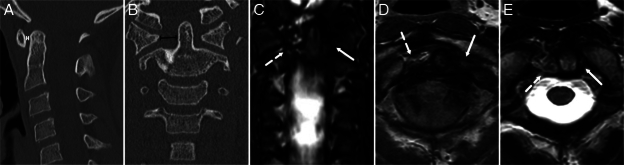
A 10-year-old male was injured in a contact sport and reported slight neck pain in the level C4-C5. The atlantodental interval was reported as normal (1.5 mm, capped white line), but the right-sided odontoid-lateral mass interval was widened (8.9 mm, capped black line). On magnetic resonance imaging (MRI), right-sided edema is seen around the odontoid [image (C), broken white arrow]. The right alar ligament was torn [image (D), broken white arrow], and the transverse ligament was partially ruptured on the right side [image (E), white broken arrow]. Solid white arrows on images (C), (D), and (E) annotate intact structures on the opposite side of the injury. (A) Noncontrast computed tomography (CT), sagittal plane, (B) Noncontrast CT, coronal plane, (C) MRI, short tau inversion recovery, coronal plane, (D) MRI, proton density, axial plane, and (E) MRI, T2-weighted, axial plane.
